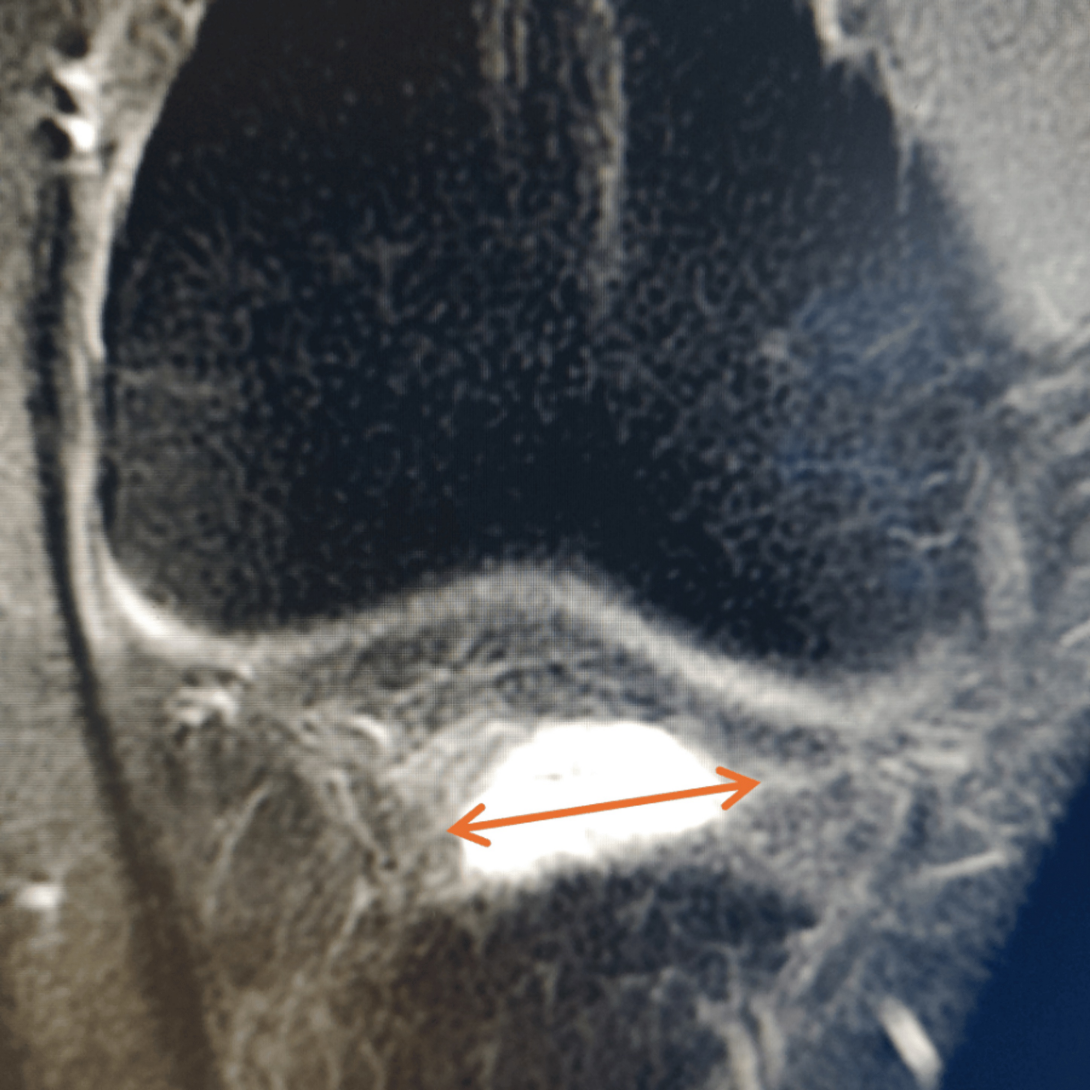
Coronal T2-weighted MRI demonstrating a ganglion cyst within Hoffa’s fat pad, anterior to the proximal tibia (arrow).
